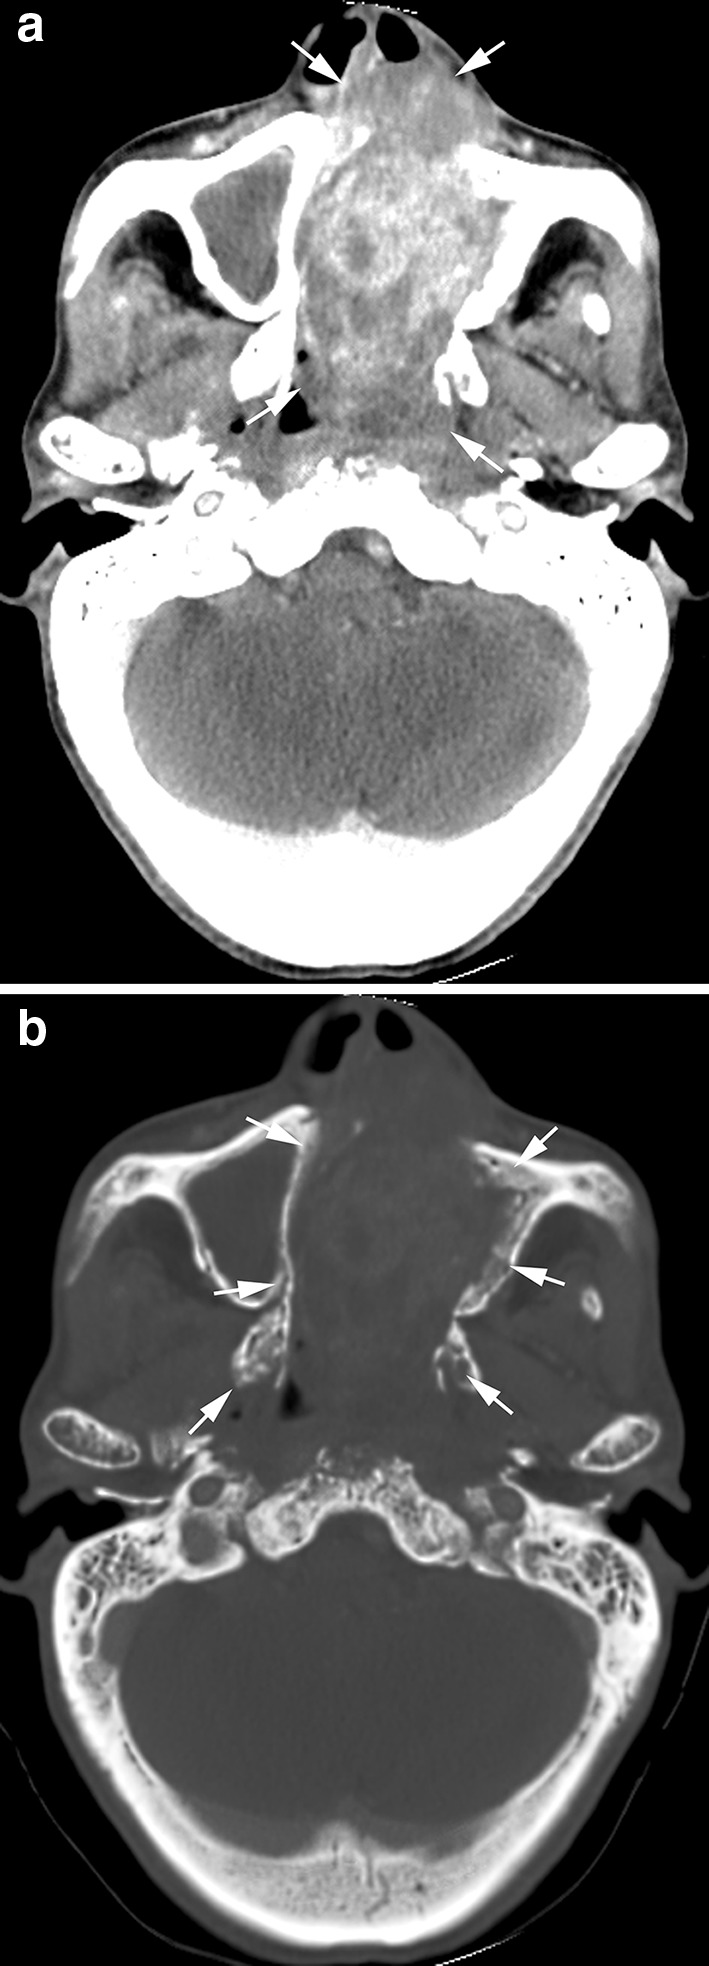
Radiation-induced undifferentiated pleomorphic sarcoma 13 years after radiotherapy of a 40-year-old man. Axial contrast-enhanced CT image (a) showed a large heterogeneously marked enhanced tumor in the nasal cavity and left maxillary sinus (*arrows*); axial CT on bony window (b) of bone destruction
